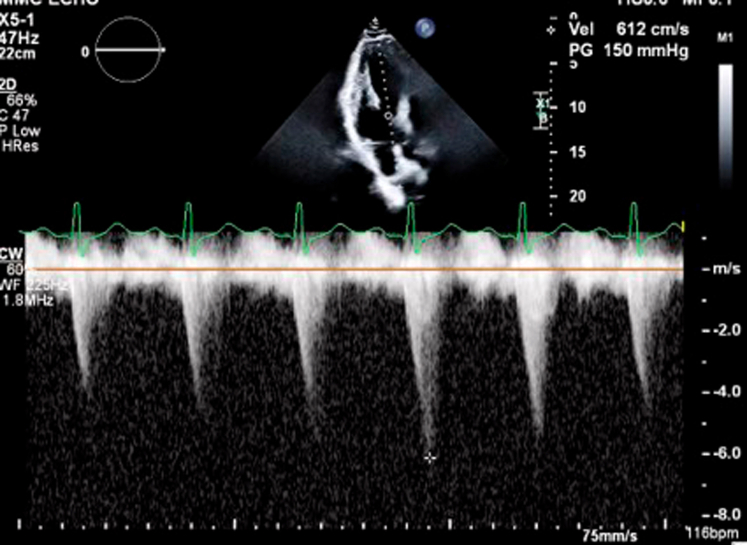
Athlete's Stress Left Ventricular Outflow Tract Gradient Continuous wave Doppler across the aortic valve during stress displaying late peaking dagger signal and significant left ventricular outflow tract obstruction with peak gradient near 150 mm Hg.
